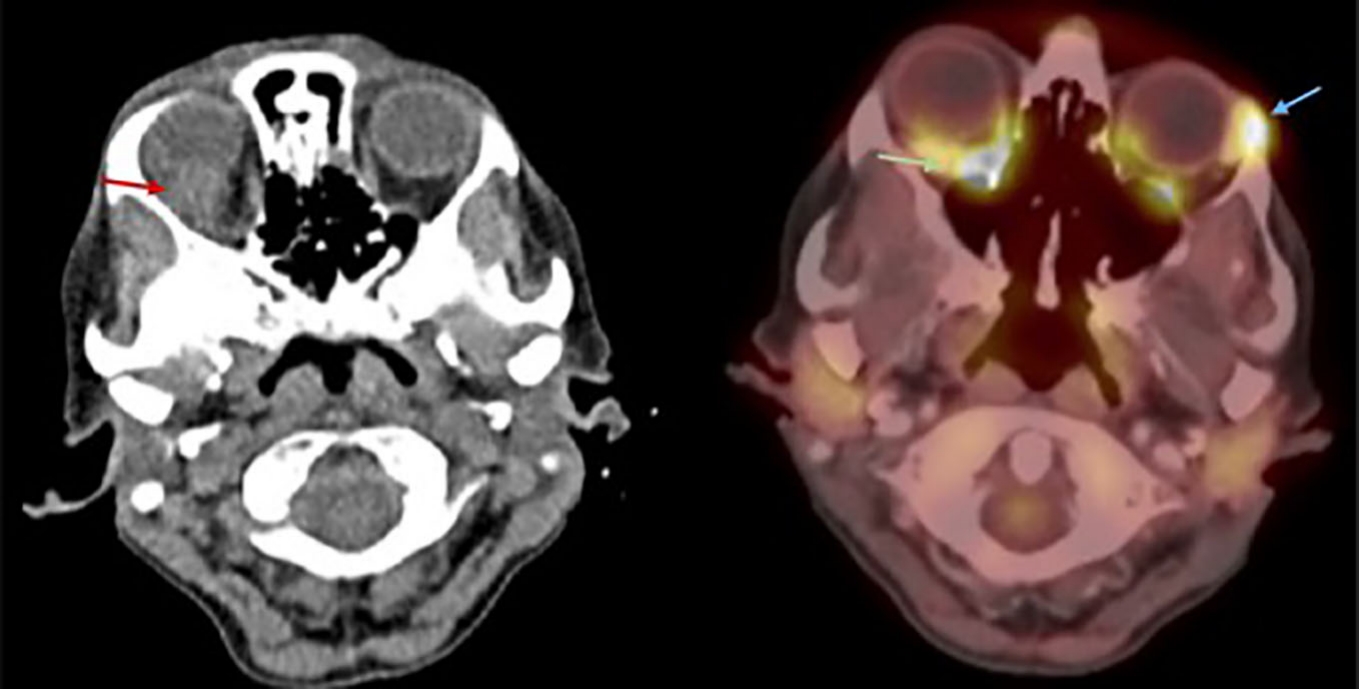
Axial MRI and PET-CT. Red arrow: Right orbital mass in the superior-temporal quadrant. Green arrow: Right orbital mass with increased FDG uptake. Blue arrow: Surgical bed after left orbital biopsy with high FDG uptake, possibly related to postsurgical inflammation.
